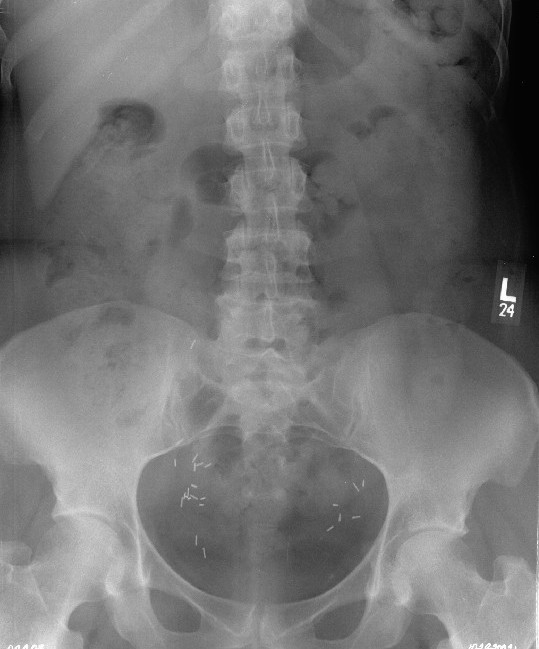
AP lumbar spine radiograph for patient #1. This film was interpreted as normal.
